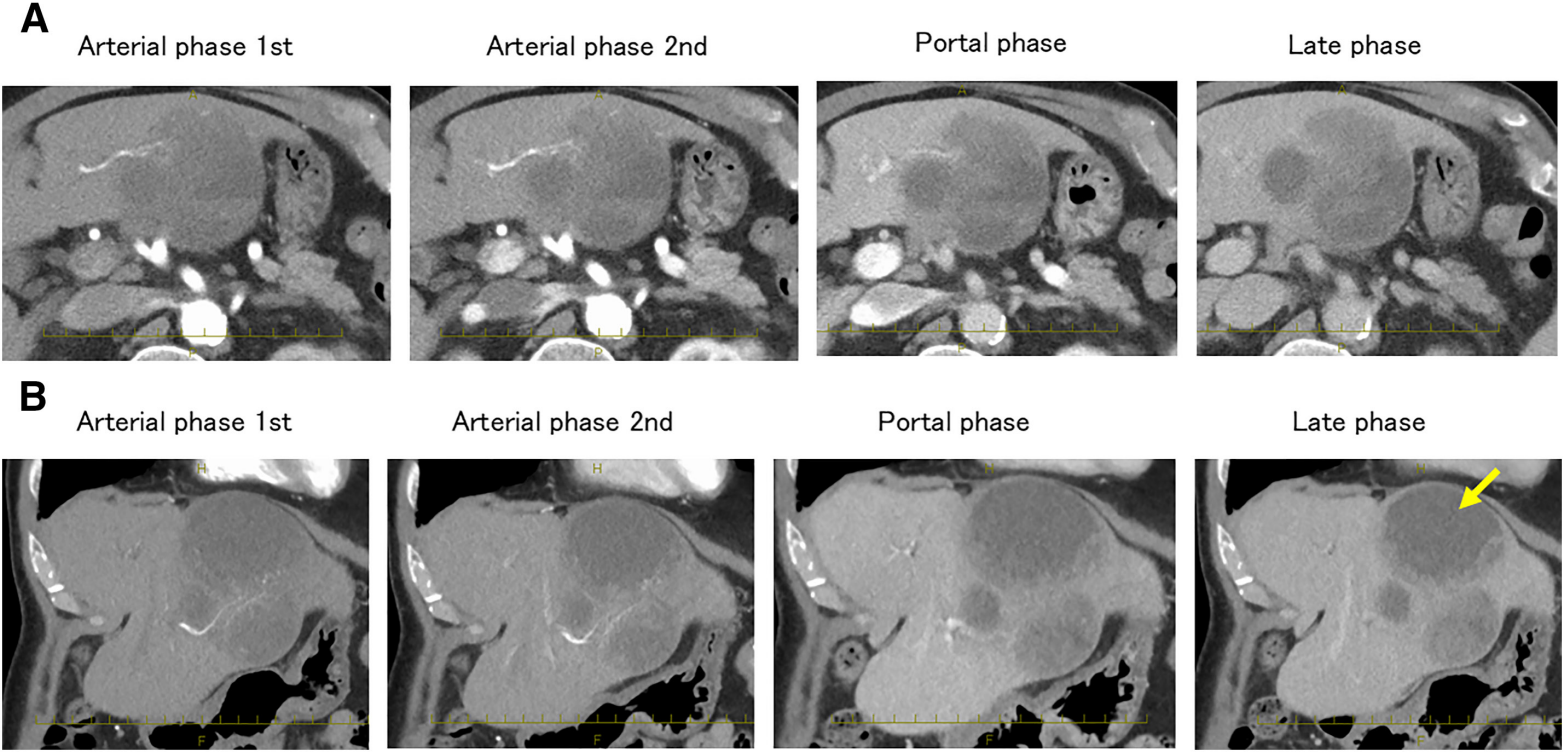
Abdominal CT findings for the tumor (A). Panel (A) shows axial 4-phase dynamic contrast-enhanced CT images of the tumor. The solid component shows contrast effects during the first and second arterial phases. In the portal and delayed phases, the contrast effect is spread over a relatively large area within the tumor. The border of the tumor is relatively clear (B). Panel (B) shows coronal CT images of the tumor. A solid tumor component is seen on the foot side of the tumor, while a liquid component (yellow arrow) is observed on the head side.
